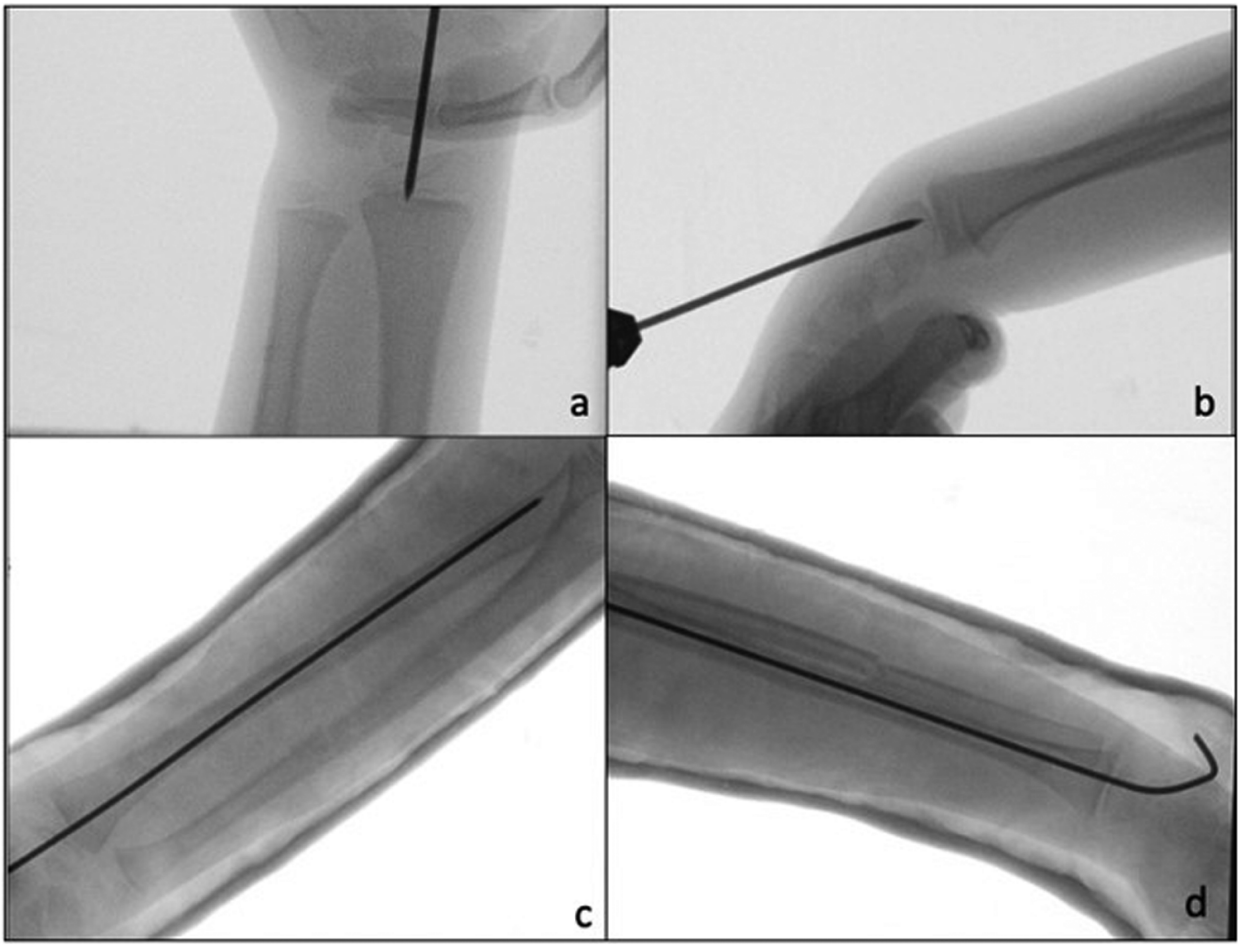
(a,b) Intraoperative fluoroscopic images show retrograde pinning through the radio-carpal joint. (c,d) Post-operative x-ray A/P and lateral, after plaster cast application.
